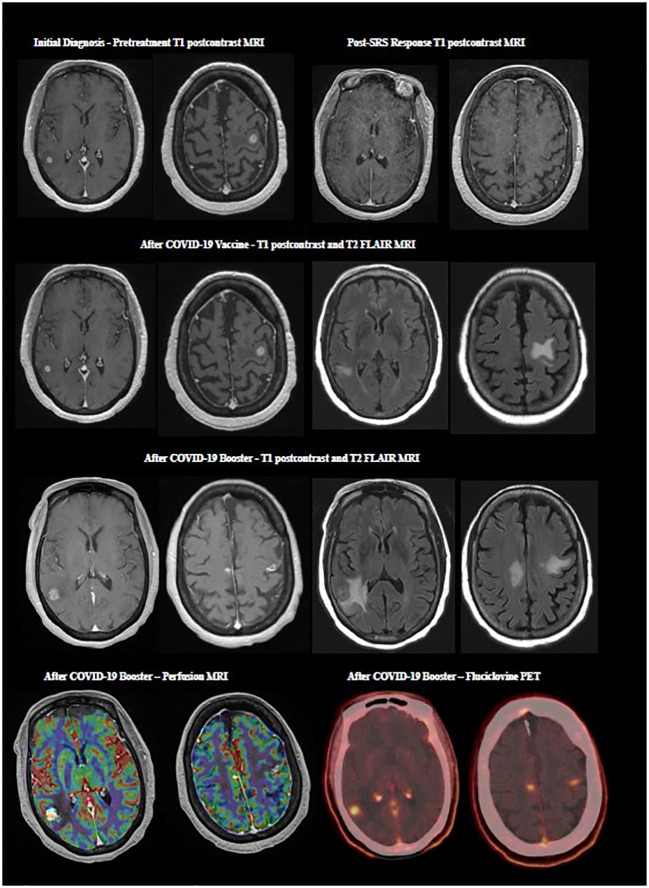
Longitudinal multimodal imaging evolution following stereotactic radiosurgery (SRS) for brain metastases. Pretreatment T1 postcontrast MRI demonstrated multiple enhancing intracranial metastases at initial diagnosis. Early post-SRS MRI showed near-complete intracranial response with minimal residual enhancement. Following COVID-19 vaccination and subsequent booster vaccinations, serial T1 postcontrast and T2 FLAIR MRI examinations demonstrated progressive enhancement, evolving edema, and treatment-related changes. Perfusion MRI demonstrated persistently decreased perfusion favoring radiation necrosis,while subsequent fluciclovine PET demonstrated hypermetabolic uptake concerning for recurrent di sease.
